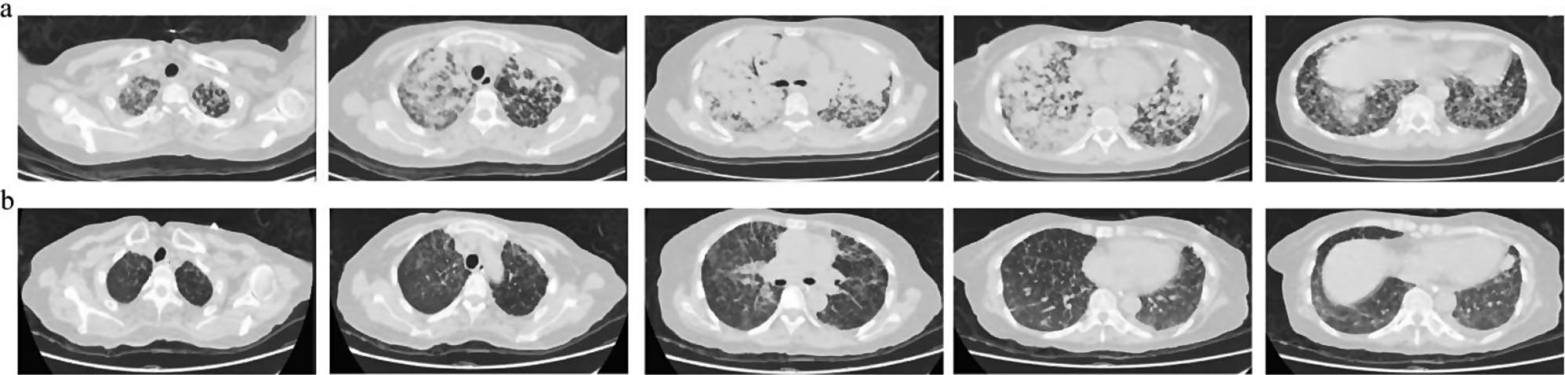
Significant improvement in chest computed tomography (CT) findings before and after treatment. (a) Multiple nodules and plaques in both lungs at initial hospitalization on January 11, 2024; (b) Resolution of diffuse nodular and patchy densification shadows in both lungs on January 30, 2024
